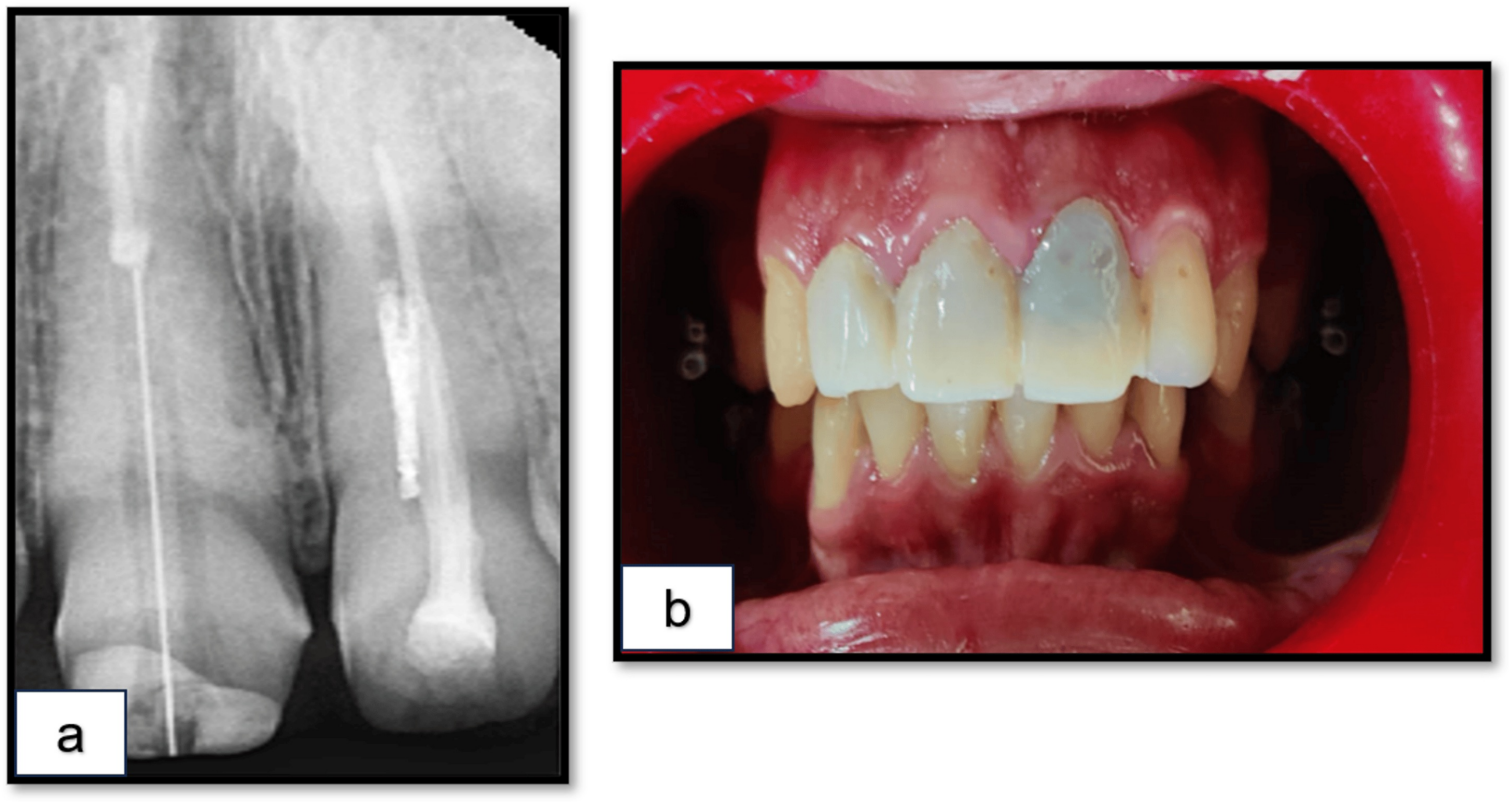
Preoperative findings at T=0 (initial presentation). (a) Radiograph showing a separated instrument in relation to tooth 21. (b) Clinical image displaying discoloration in relation to tooth 21.
